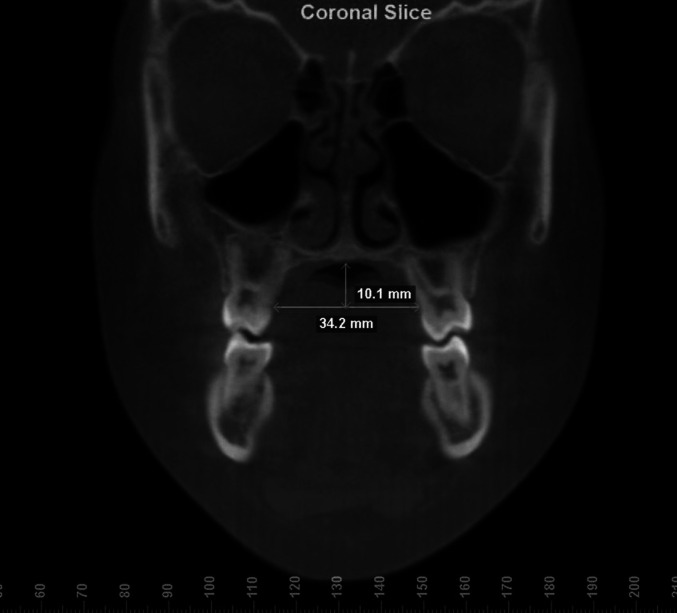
Width and depth hard palate measurements at first molar level with cone-beam computed tomography scan, coronal plane.
